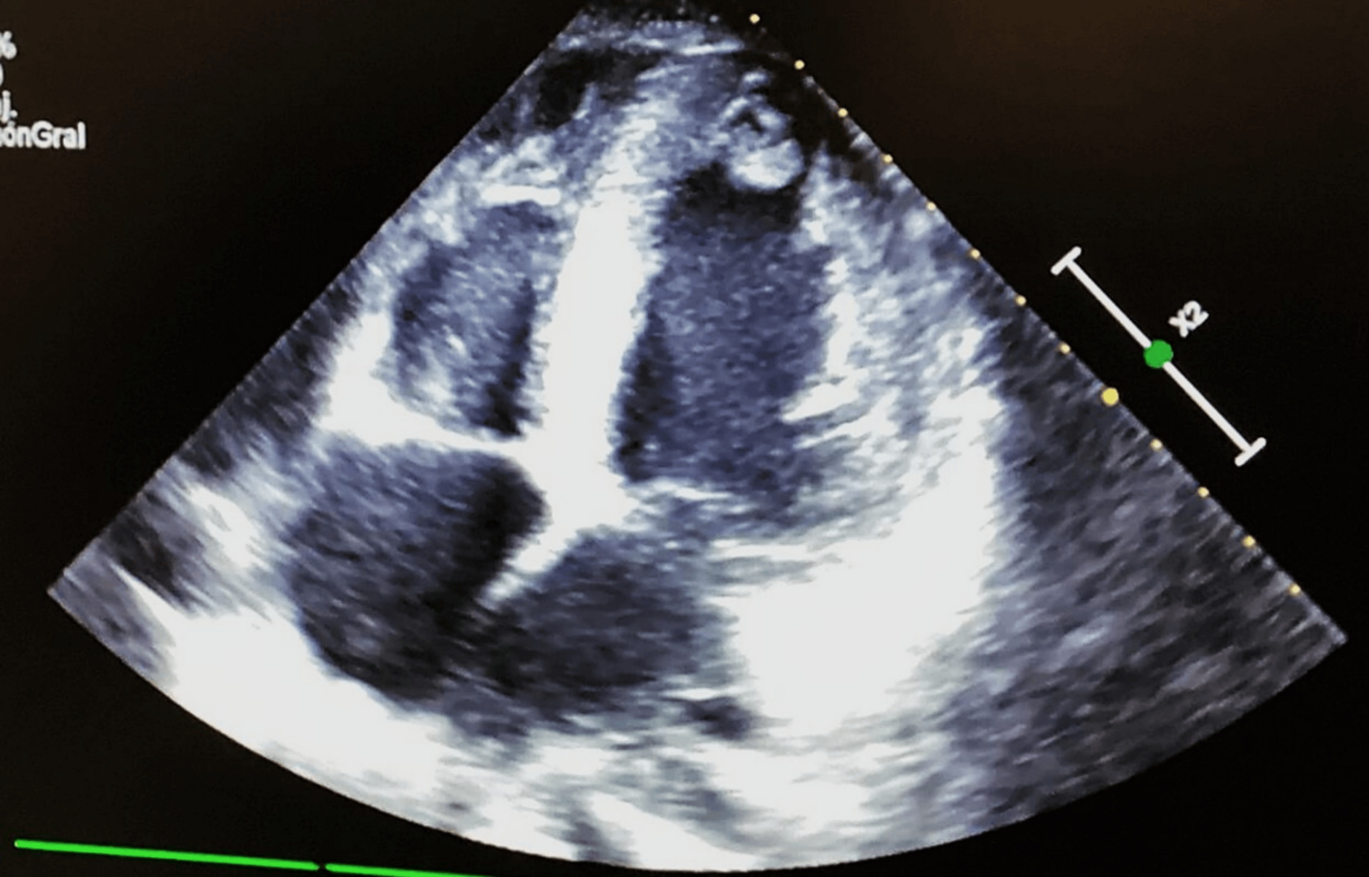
Echocardiogram Biventricular failure with a left ventricular ejection fraction (LVEF) of 33%, concentric hypertrophy without signs of pulmonary hypertension, and systolic and diastolic dysfunction of both ventricles. An intracavitary thrombus was observed in the left ventricle, measuring 2.5 × 2 cm, along with dilation of both the right and left chambers. The right atrial systolic pressure (RASP) was 3 mmHg.
